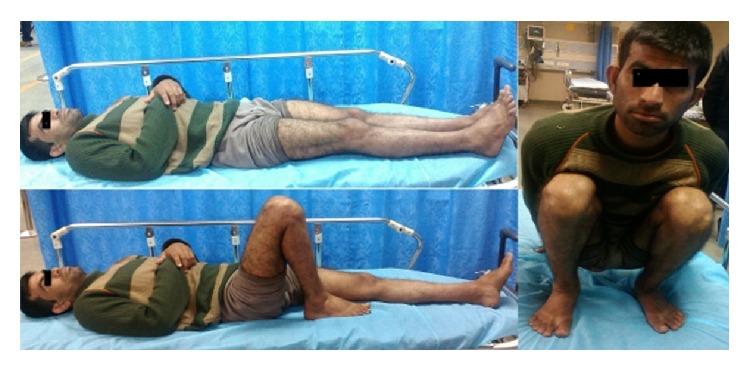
Image showing movement at knee joint in a high energy tibial plateau fracture (radiograph depicted as in Figure 1(a)).
